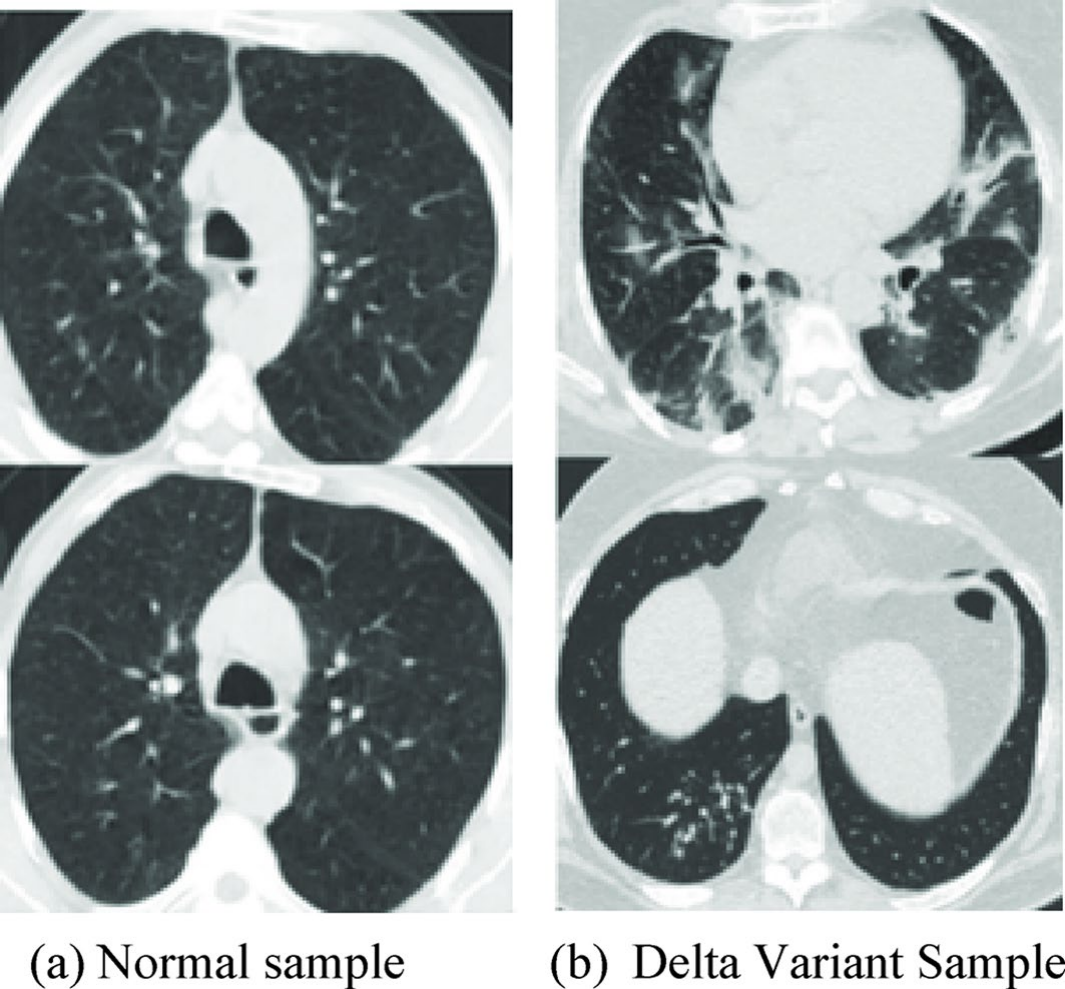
CT images for Normal and Delta variant samples.
